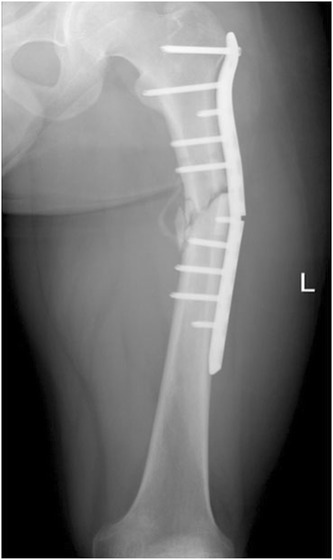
The anteroposterior radiograph of proximal femur shows the plate breakage and nonunited proximal femoral shaft fracture
